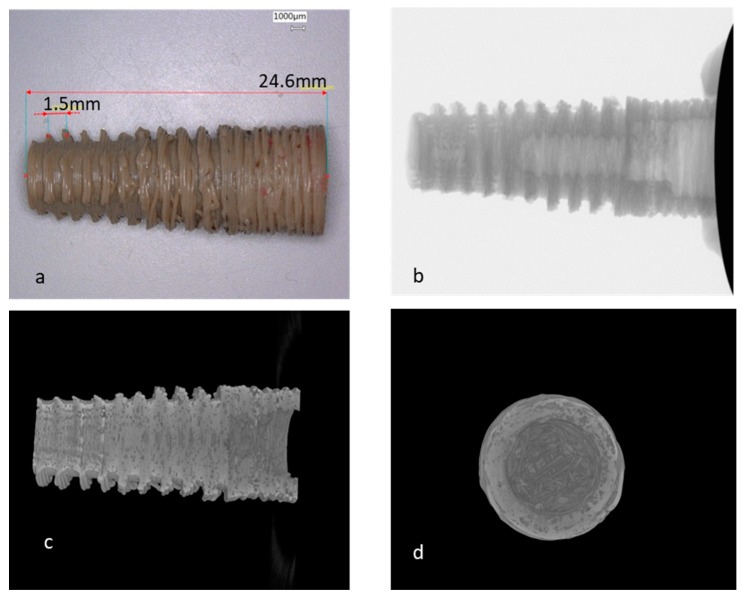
CT of a magnified dental implant produced with Apium HPP155 ((a) is the printed implant, (b–d) are micro CTs of the sample).
